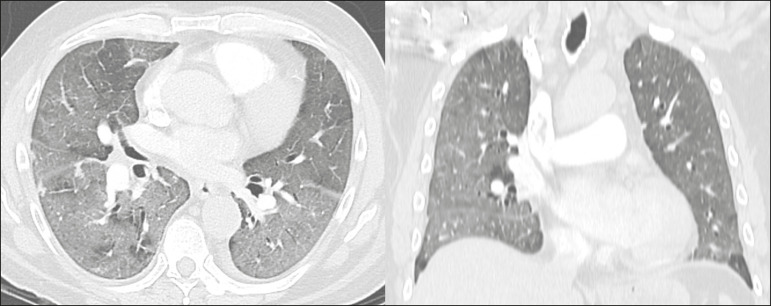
Example of indeterminate findings for COVID-19 on chest CT, showing bilateral diffuse ground-glass opacities with atypical distribution. RT-PCR test was positive for rinovirus.
